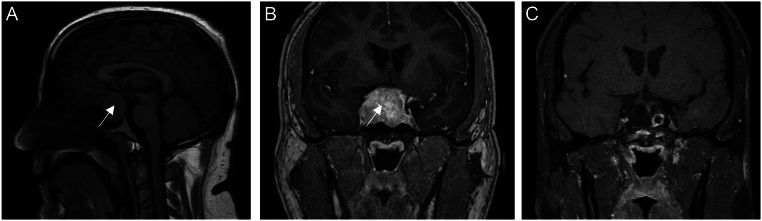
(A and B) MR imaging pre-operatively in the sagittal and coronal planes demonstrated a 3.0 × 2.5 × 3.0 cm sellar and suprasellar region mass. There is optic chiasm compression. No other intracranial pathology was noted. (C) Post-operative pituitary imaging demonstrates no evidence of residual disease or recurrence.
